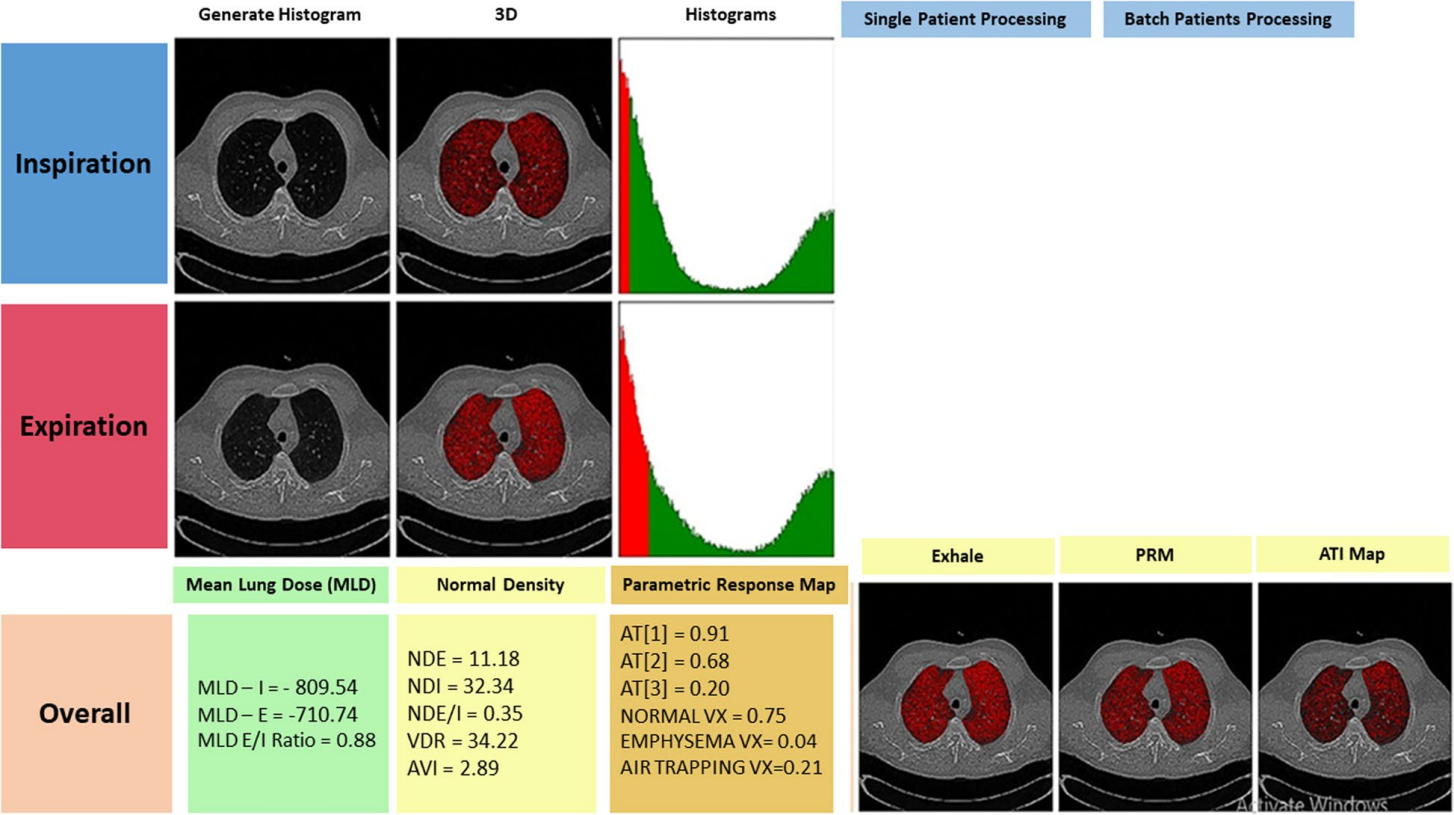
Example of a CT scan of a patient interpreted by the BLADQA
